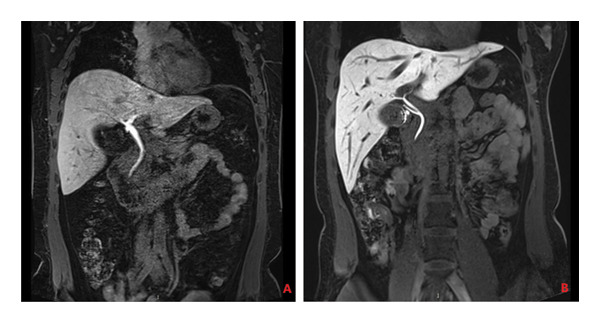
(A) Gd‐EOB‐DTPA‐enhanced MRI hepatobiliary phase image of a fasting 40‐year‐old female patient, showing a turbid cystic duct and impeded bile flow into the gallbladder. (B) Gd‐EOB‐DTPA‐enhanced MRI hepatobiliary phase image of a fasting 36‐year‐old female patient, showing clear bile flow into the gallbladder.
